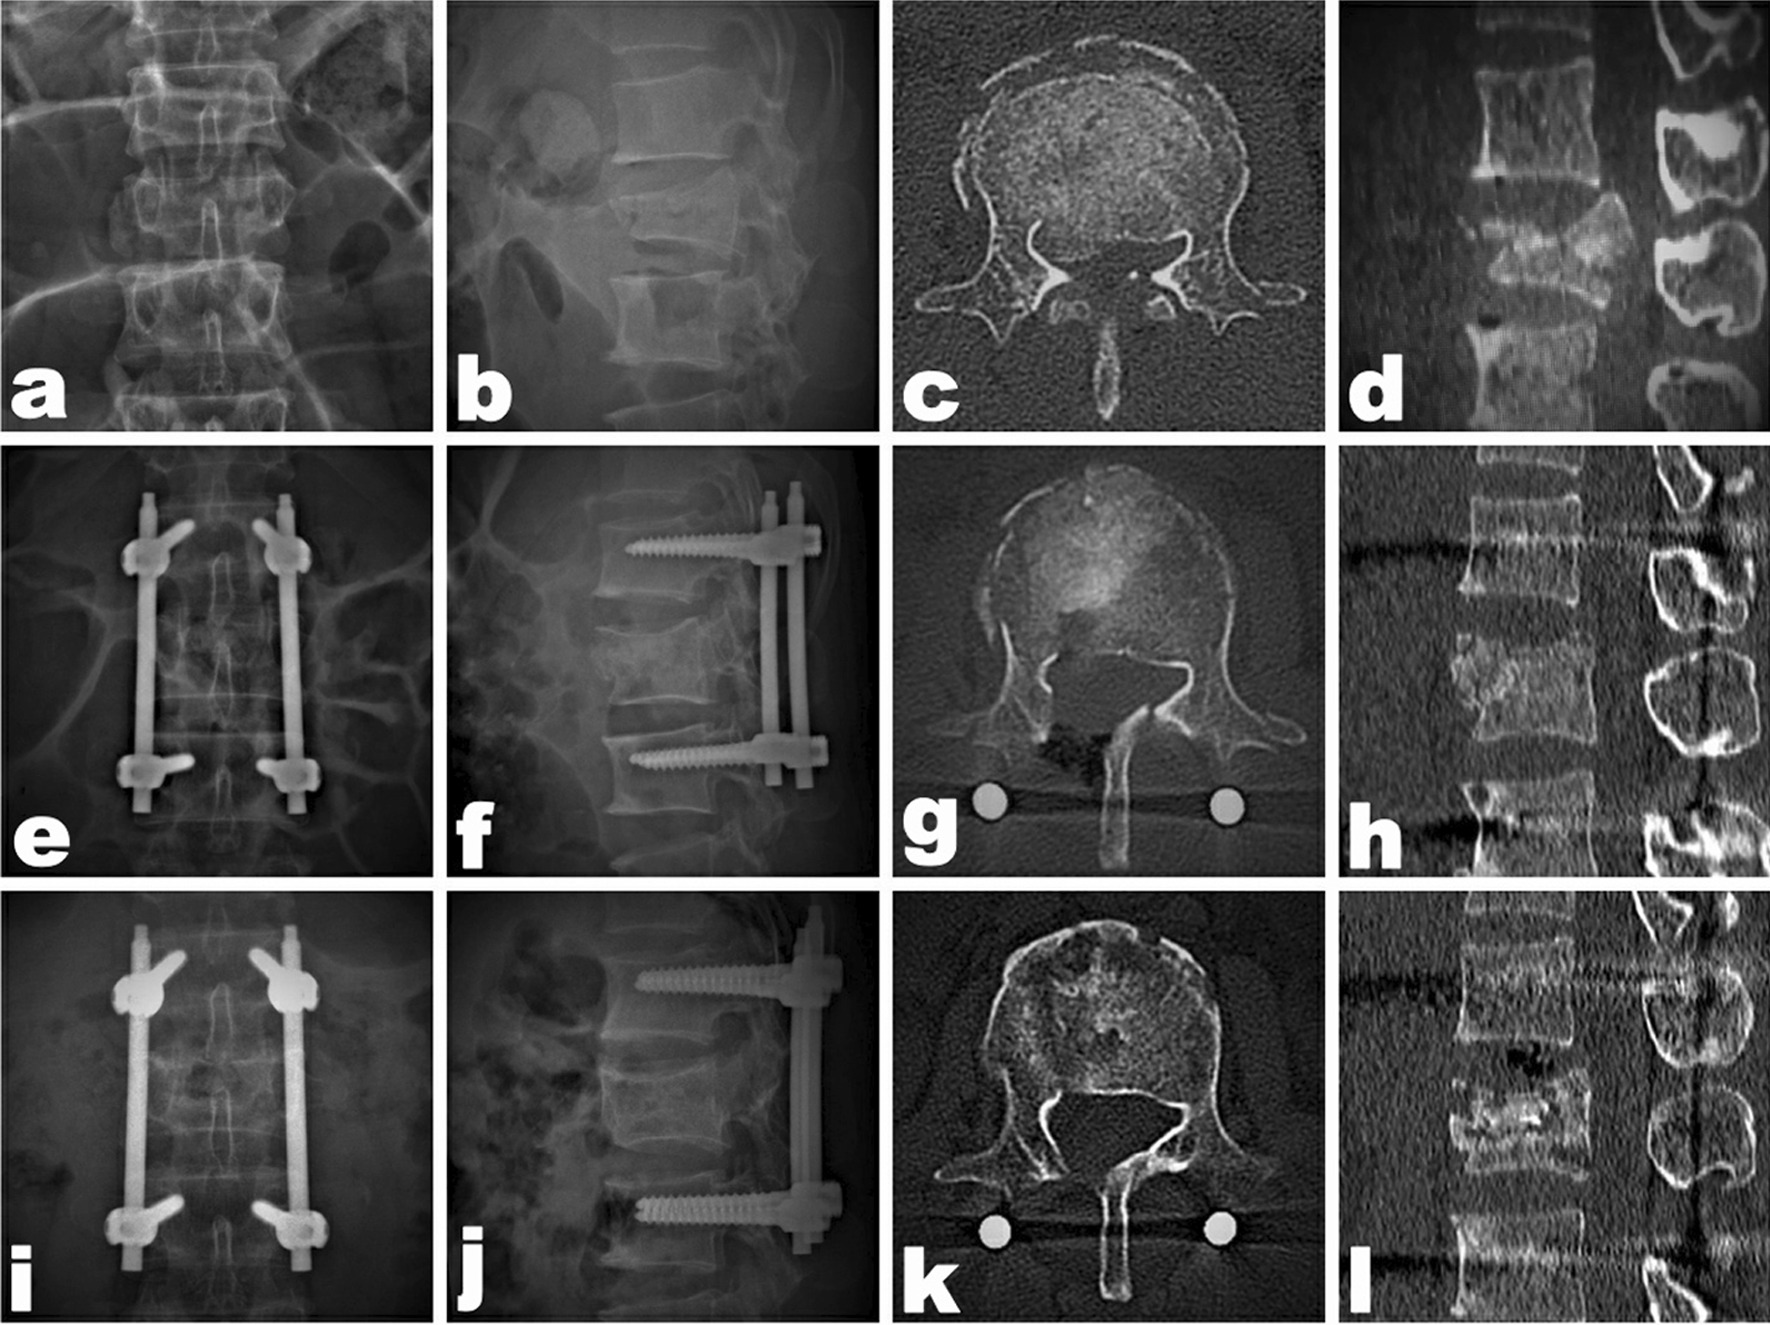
A 47-year-old man with L2 burst fracture (AO type A4) with severe traumatic spinal stenosis. a Preoperative anteroposterior radiograph; b preoperative lateral radiograph; c preoperative CT axial view; d preoperative CT sagittal view; e postoperative anteroposterior radiograph; f postoperative lateral radiograph; g postoperative CT axial view; h postoperative CT sagittal view; i 2-year postoperative anteroposterior radiograph; j 2-year postoperative lateral radiograph; k 2-year postoperative CT axial view; and l 2-year postoperative CT sagittal view
